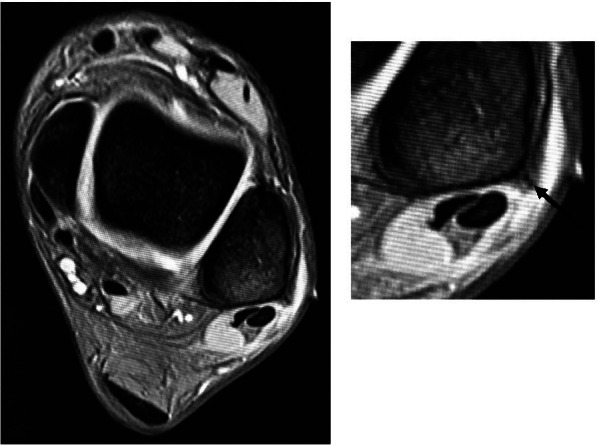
Preoperative MRI (T2WI). MRI showing a formed false-pouch, although the fibrocartilaginous ridge (arrow) remains intact
